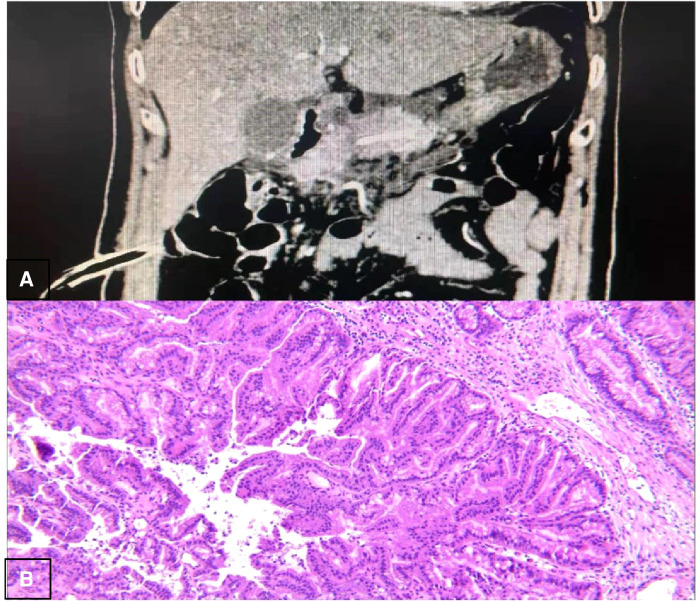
Postoperative outcome: CT and pathological images. A: CT images, B: pathological images.
